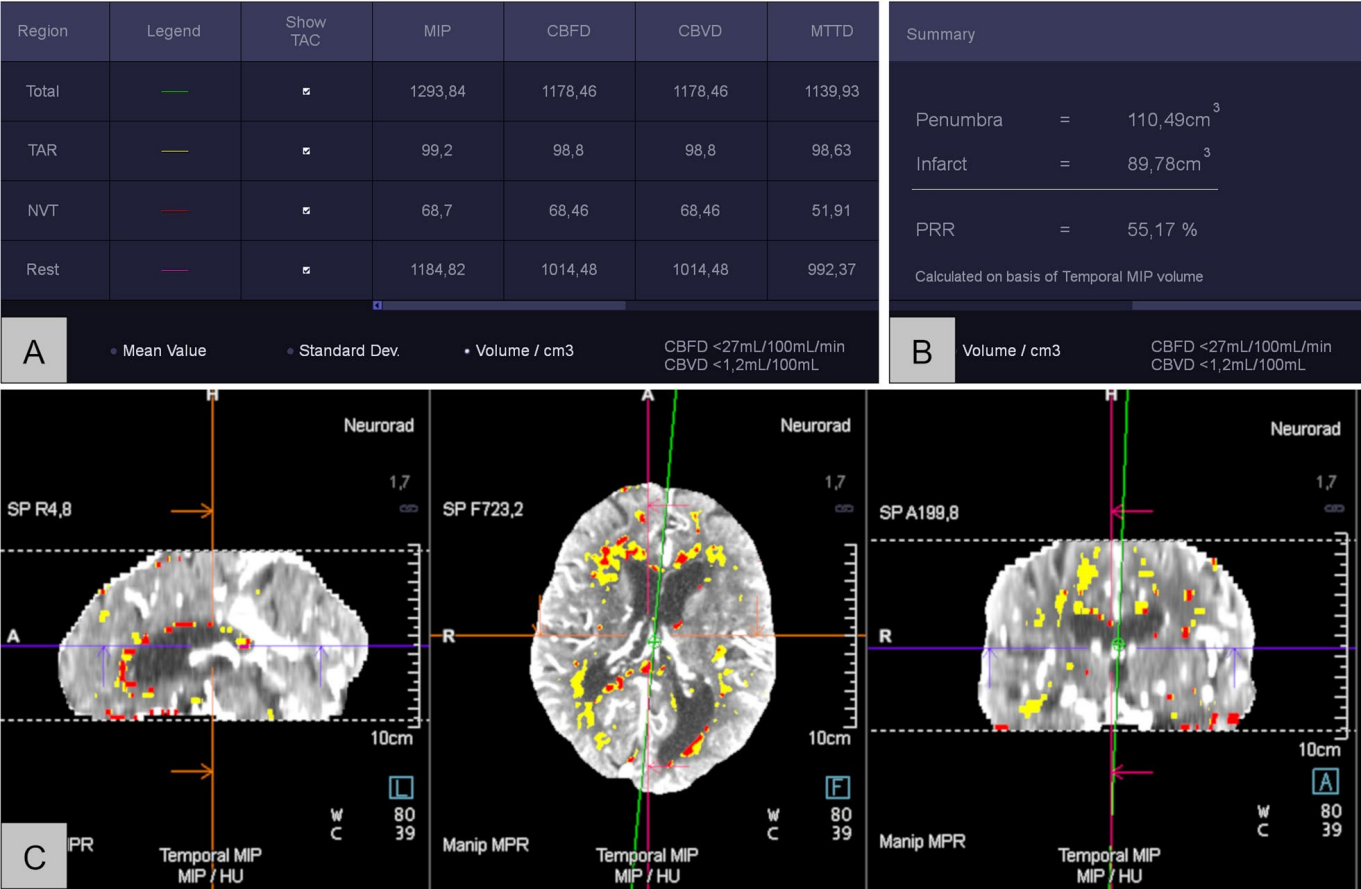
Screenshots of the Syngo.via Software. A Collecting the volumes of MIP/CBF/CBV and MTT, B penumbra and Infarct volume acquisition, C topogram of CTP. *MIP* maximum intensity projection, *CBF* cerebral blood flow, *CBV* cerebral blood volume, *MTT* mean transit time, *CTP* computed tomography perfusion, *TAR* tissue at risk, *NVT* non-viable tissue, *TAC* time attenuation curve, *PRR* penumbra relative ratio, *HU* Hounsfield unit, *MPR* multiplane reconstruction
